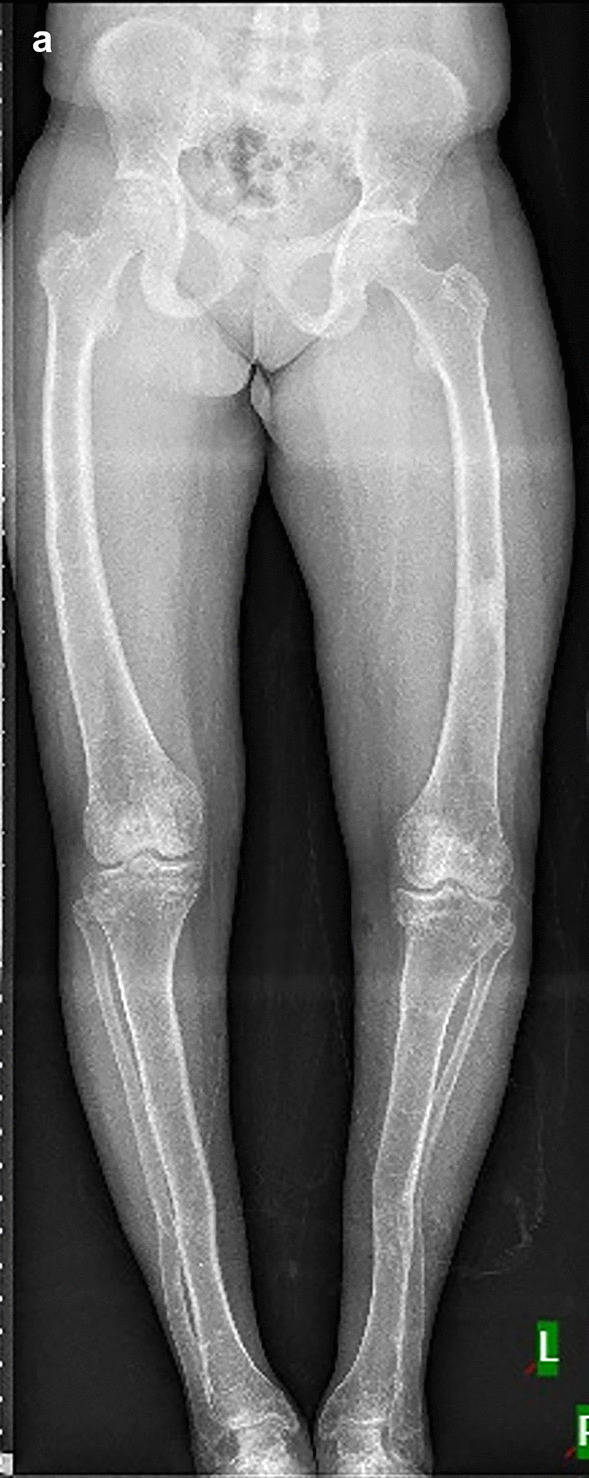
Lower limb radiograph of P6 with X-linked hypophosphatemic rickets (age 22 y). Bowing of long bones, and osteopenia with frayed metaphyses are seen (a)
